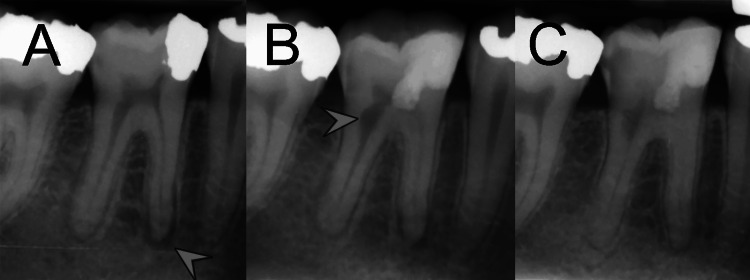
Radiographic progression of the treated case (A) Preoperative periapical radiograph showing a deep amalgam restoration close to the pulp in the lower right first molar, with an apical lesion in the mesial root (arrowhead), PDL widening in the distal root, and large root canals, particularly in the distal root. (B) Immediate postoperative radiograph after partial pulpotomy with CEM cement, revealing an IRR lesion (arrowhead) that developed during the month following the initial diagnosis. (C) Radiograph at 24-month follow-up demonstrates complete apical healing, recalcification of the IRR lesion, narrowing of the root canals, and normal PDL space, with no signs of further pathology. The tooth remained functional and asymptomatic, confirming the successful treatment outcome. PDL, periodontal ligament; CEM, calcium-enriched mixture; IRR, internal root resorption
